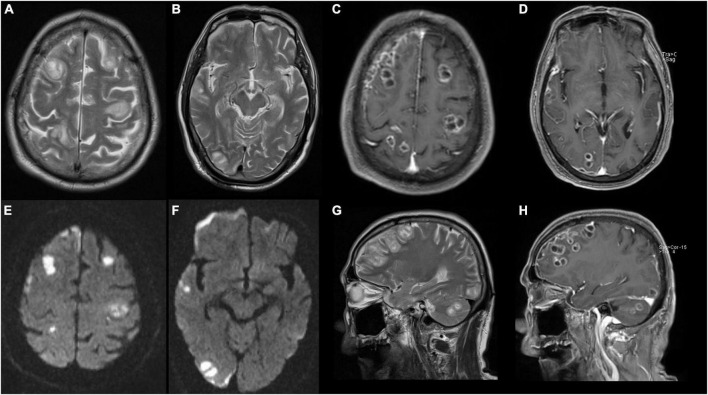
Magnetic resonance image (MRI) of brain shows abscesses in bilateral frontal lobe, right temporo-parieto-occipital lobe, and right cerebellar hemisphere with ring contrast enhancement and perilesional edema. (A,B,G) T2-weighted imaging. (C,D,H) T2 contrast-enhancement imaging. (E,F) Diffusion-weighted MRI.
